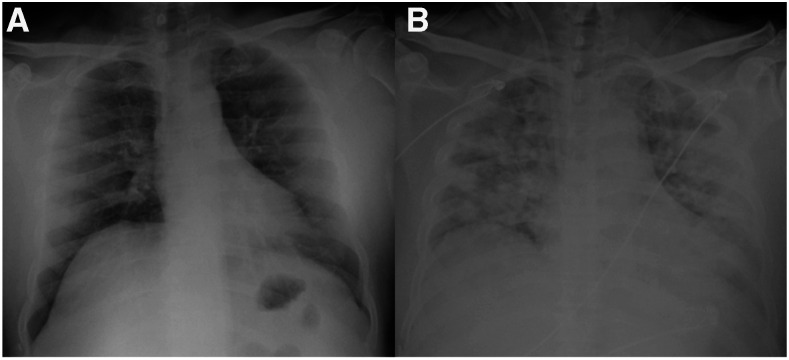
Radiographic evolution. (A) Chest X-ray at hospitalization showing bilateral ground-glass opacities with peripheral predominance. (B) Chest X-ray on day 7 showing radiographic progression, with diffuse bilateral alveolar occupation and consolidations.
